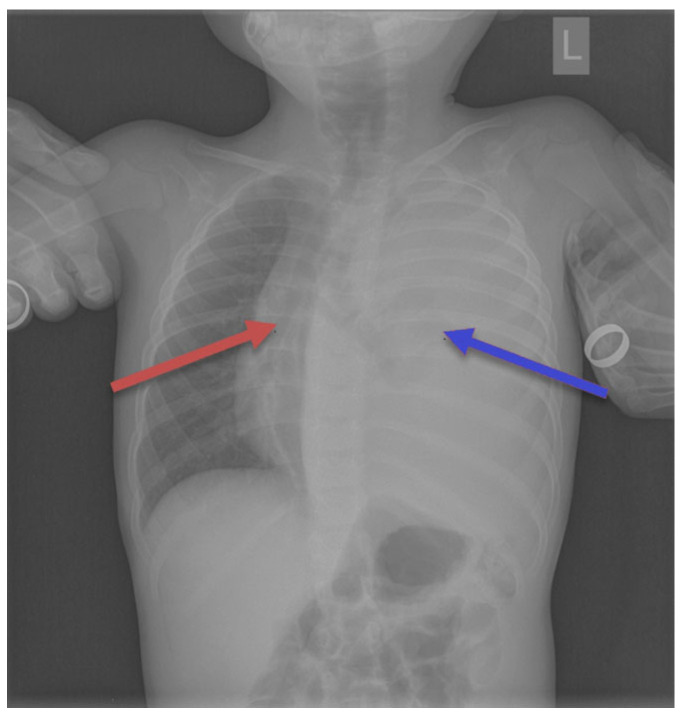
Chest radiograph showed complete homogenous opacification of the left hemithorax (the blue arrow) with contralateral mediastinal and tracheal deviation (the red arrow).
